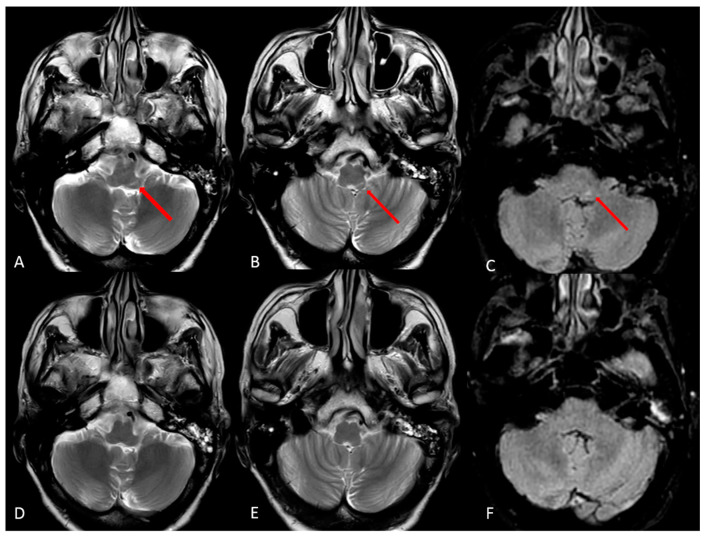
(A–C) MRI scan with contrast of the patient after the second hospitalization; (D–F) MRI scan without contrast performed during the first hospitalization. (A,B,D,F) T2-weighted images; (C,F) FLAIR images. (A–C) Hyperintense lesion (red arrows) in the posterior lower pons on the left side extended to the medulla, in the presumed side of cochlear and vestibular nuclei.
